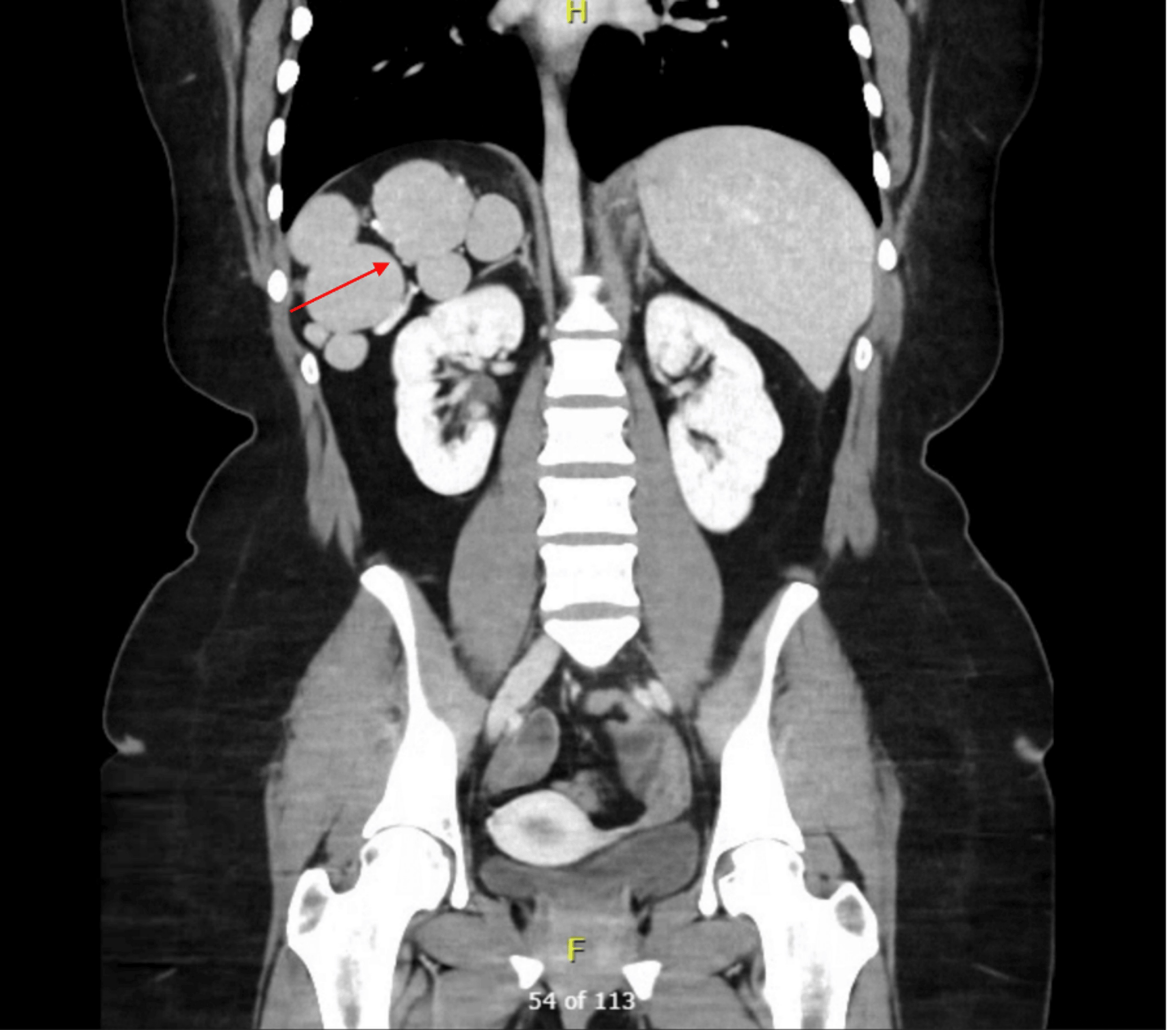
Computed tomography of the abdomen and pelvis with contrast Coronal section displaying left-sided liver, with the red arrow indicating polysplenia in the right upper quadrant.
